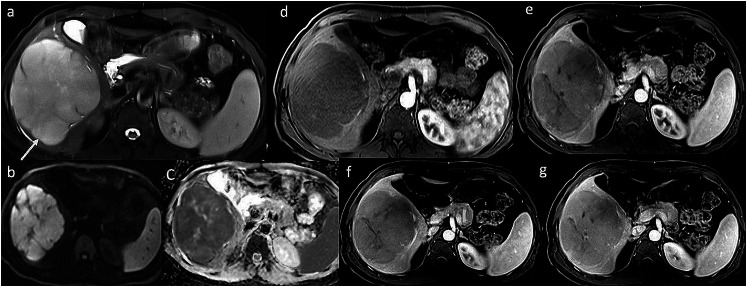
Multiphasic contrast enhanced MRI of liver. (a) Axial T2W image shows a hyperintense lesion (arrow) which depicts marked diffusion restriction on diffusion weighted image (b) and corresponding apparent diffusion coefficient (ADC) map (c) suggesting high cellularity of the lesion. Post-contrast T1W images in (d) early arterial, (e) late arterial, (f) portal venous, (g) hepatic venous phases show hypoenhancing lesion with mild progressive enhancement of the lesion
